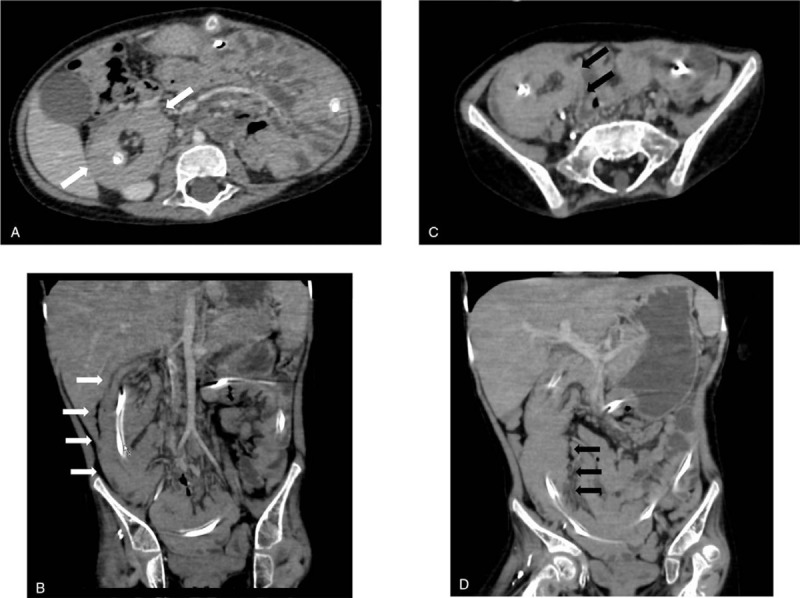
The contrast computed tomography scan showed wall thickening and intussusception of the duodenum (A, B; white arrows) and jejunum (C, D; black arrows).
